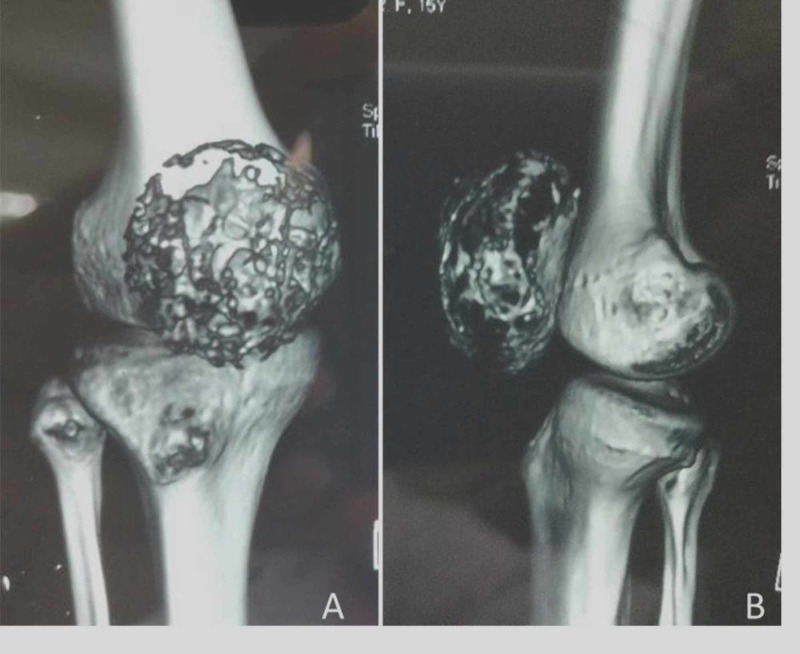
Computed tomography scan shows complete involvement of whole patella with no soft tissue extension
